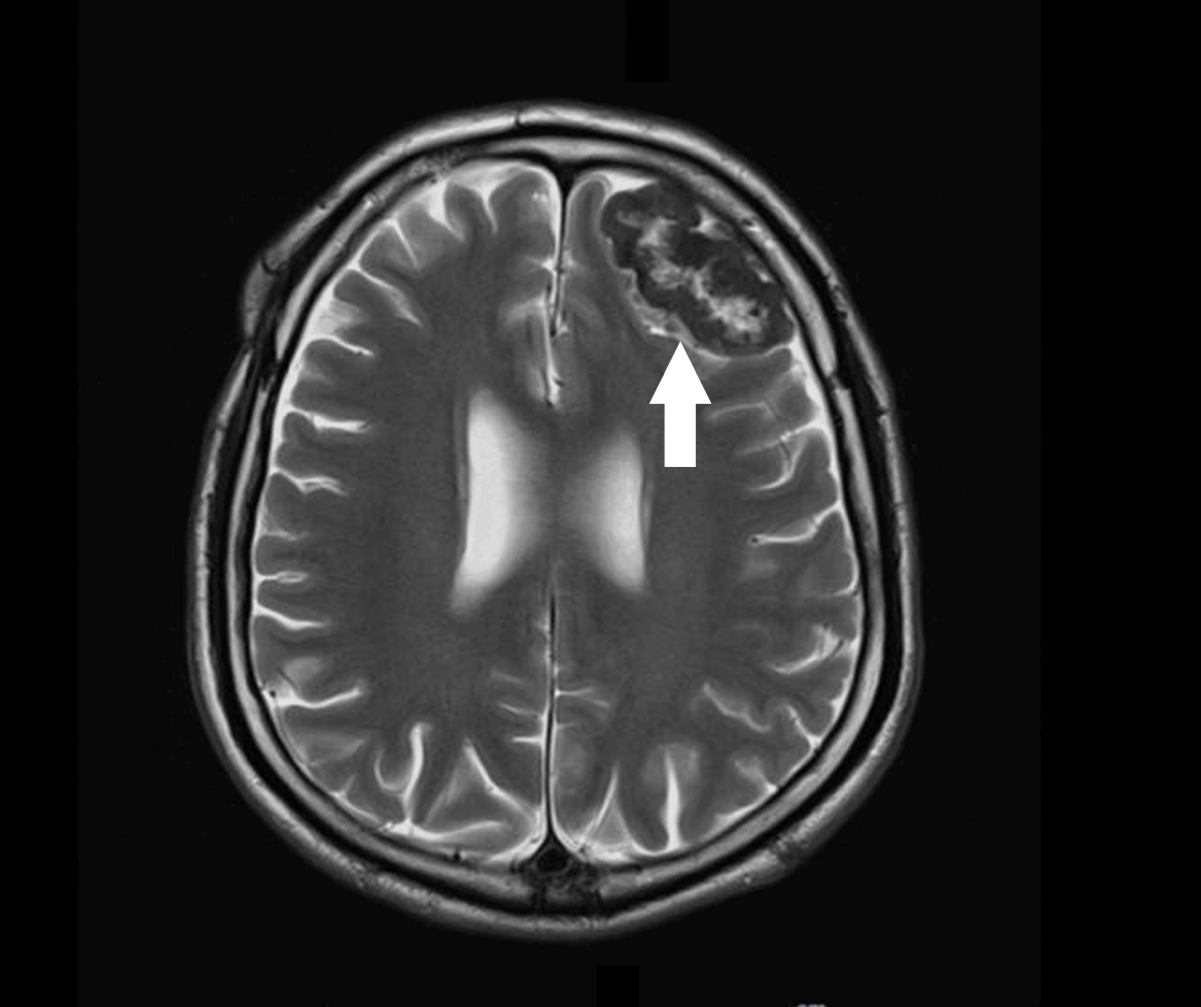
MRI brain with contrast: Axial-view T2-weighted image showing a left frontal lobe lesion
